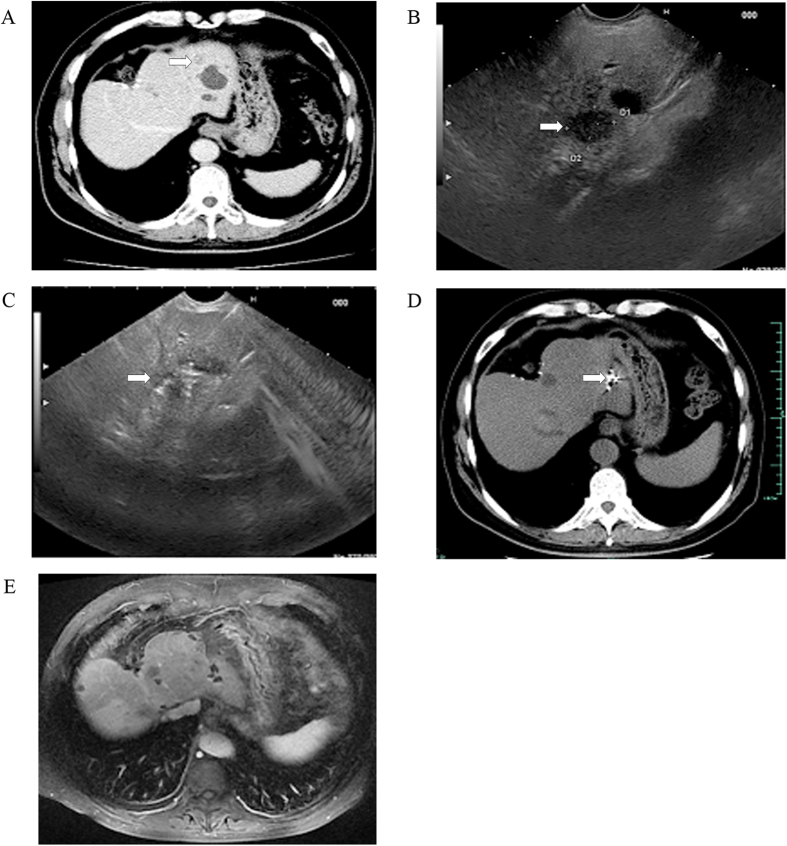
EUS-guided iodine-125 brachytherapy for postoperative liver metastasis of cholangiocarcinoma. (A) presence of a 1.3-cm, low-density liver mass located in the left lobe on CT scan (white arrows). US image failed to show the lesions due to the gas ahead; (B) identification of a 1.5 cm × 1.3 cm low-echodensity mass on EUS (white arrows); (C) EUS-guided implantation of iodine-125 particles (white arrows); (D) obvious downsizing of the liver disease on follow-up CT scan at 1 months (white arrows); and (E) disappearance of the liver disease on follow-up MRI scan taken after 12 months.
